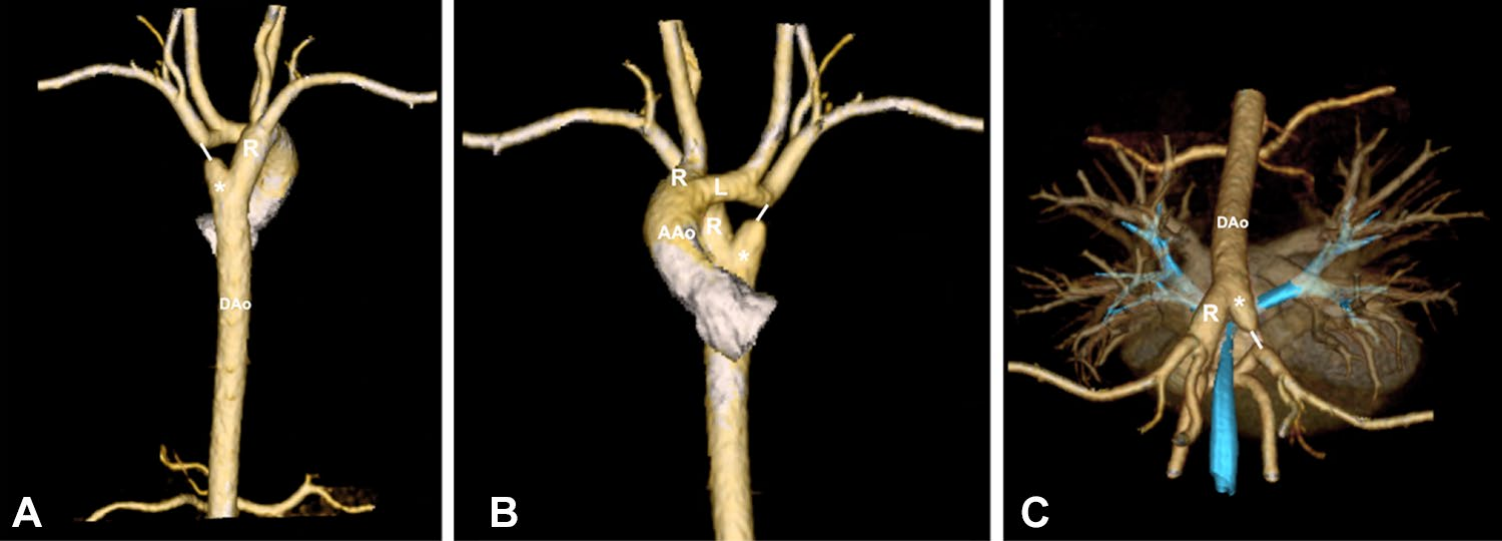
3D reconstruction of a double aortic arch. Anterior and posterior view are shown in pictures (A and B). Superior view depicts the vascular anatomy and its relationship with the trachea (C). Dao: Descending aorta. R, right aortic arch; AAo, ascending aorta; L, left aortic arch; asterisk, Kommerell diverticulum; white bar, ligamentum arteriosum (no captured by CT imaging)
